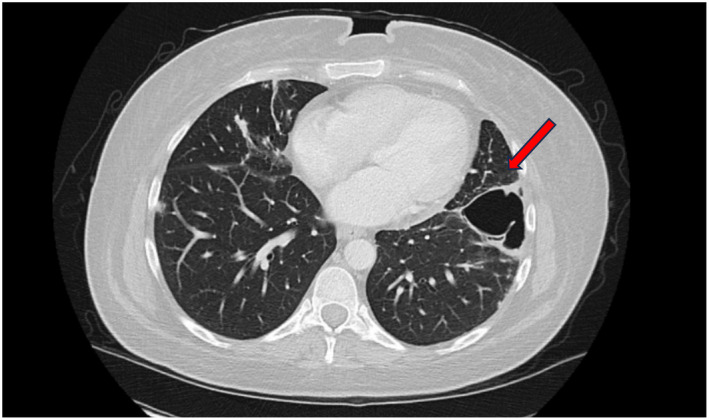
Chest CT 09/2019: Patchy areas of consolidation/nodules bilaterally improved since prior examination likely from steroid treatment. Continued cavitary within LEFT lower lobe like prior examination.
